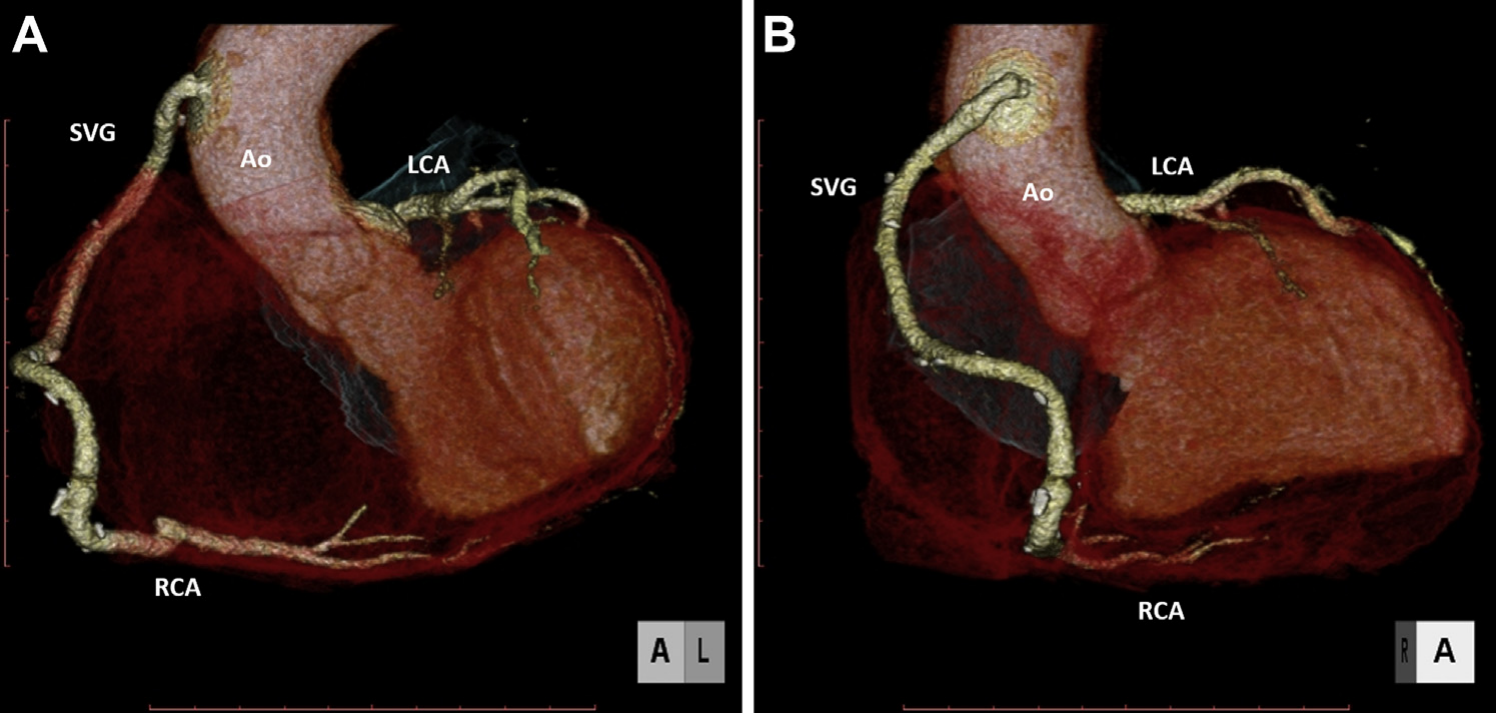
Post-Operative Enhanced Computed Tomography Imaging Pseudoaneurysm, right coronary aneurysm, and abnormal coronary fistula were completely resected. (A) Left anterior view. (B) Anterior view. SVG = saphenous vein graft; other abbreviations as in Figure 2.
